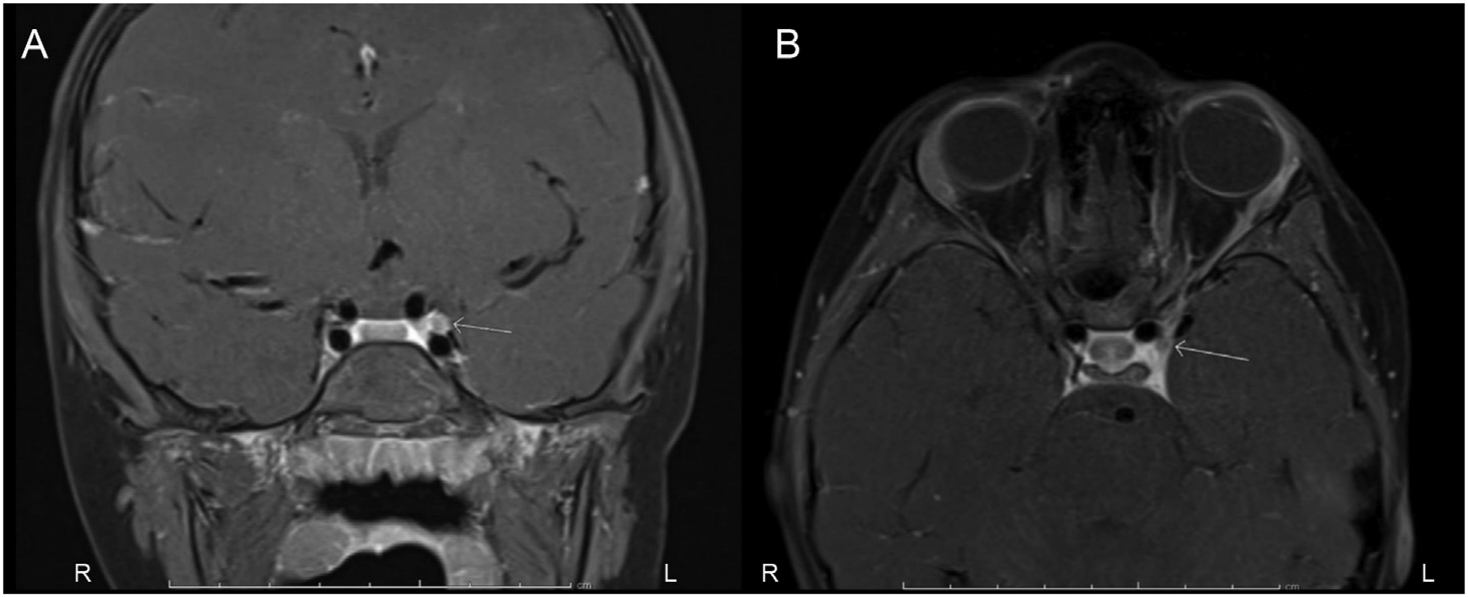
Coronal (A) and axial (B) high-resolution, fat-saturated, post-contrast T1 MRI showing a nodule of relative hypoenhancement compared to venous blood within the left cavernous sinus (arrows).
